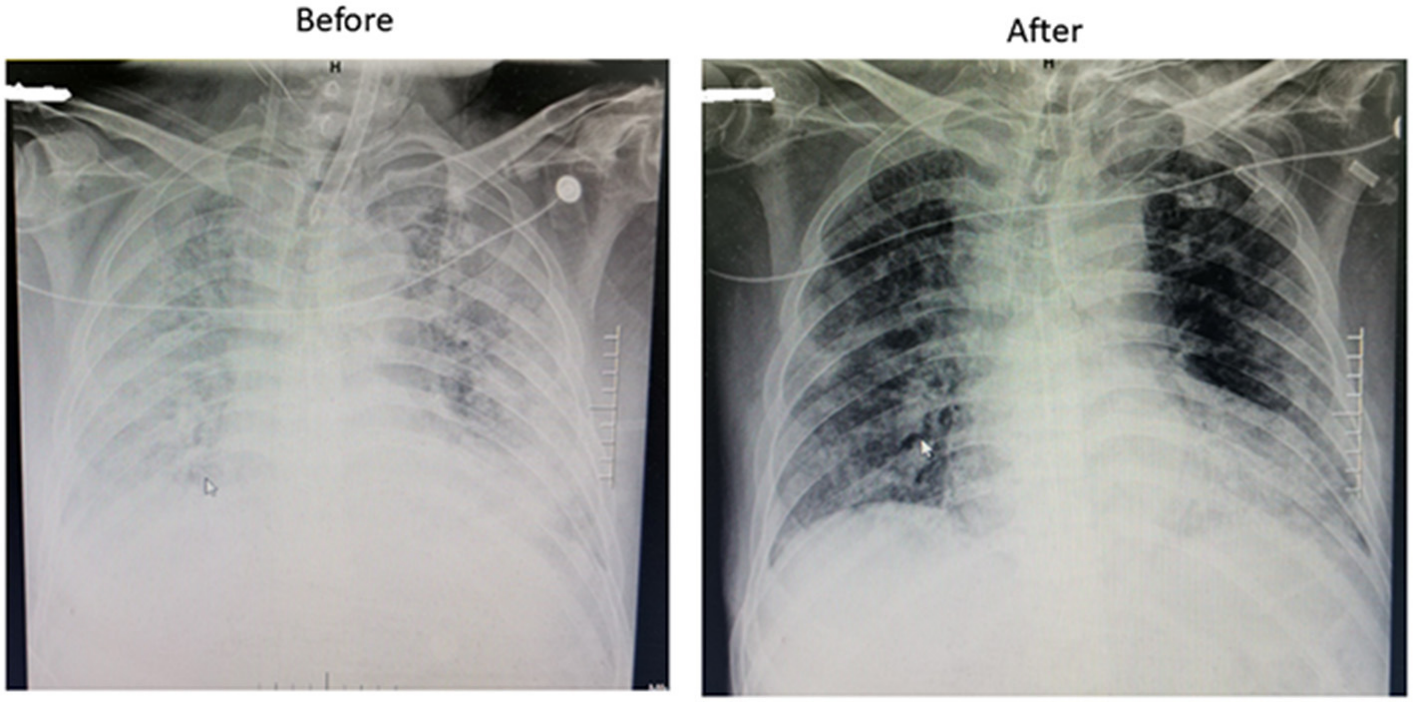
Representative chest X-ray of COVID-19 patients before and after ECMO treatment.
